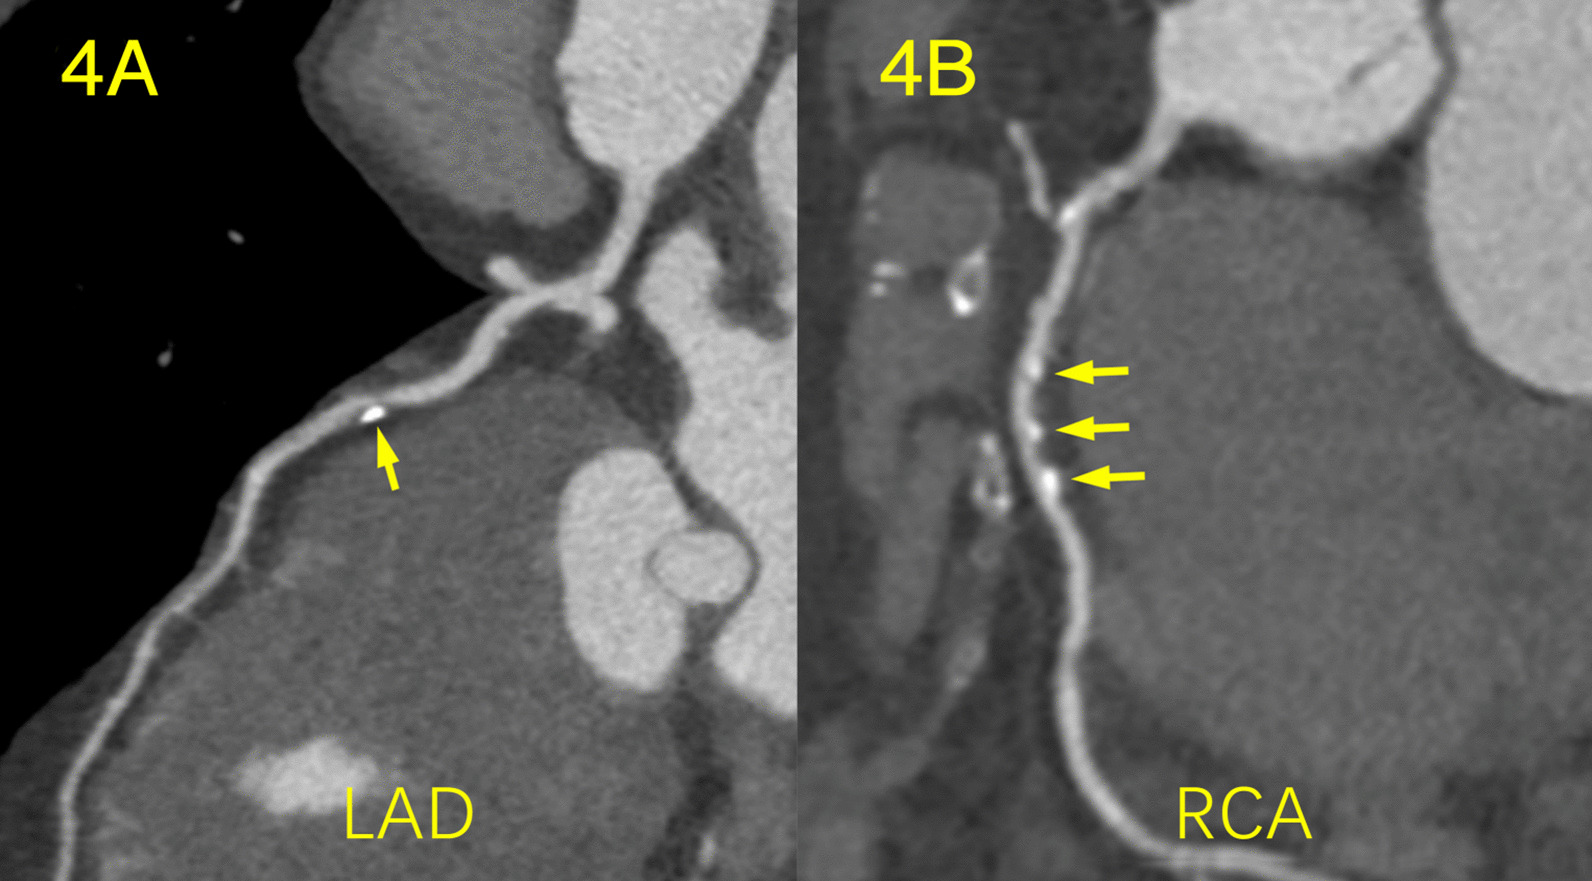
Mixed plaque in a 55-year-old man with moderate SCH and hypertension. The patient underwent coronary CTA for chest tightness. a Curvature planar reconstruction CT image of the LAD artery shows a mixed plaque in the proximal segment of the LAD with moderate stenosis. b The image of the RCA artery shows a long mixed plaque in proximal and middle segments of the RCA with moderate stenosis
